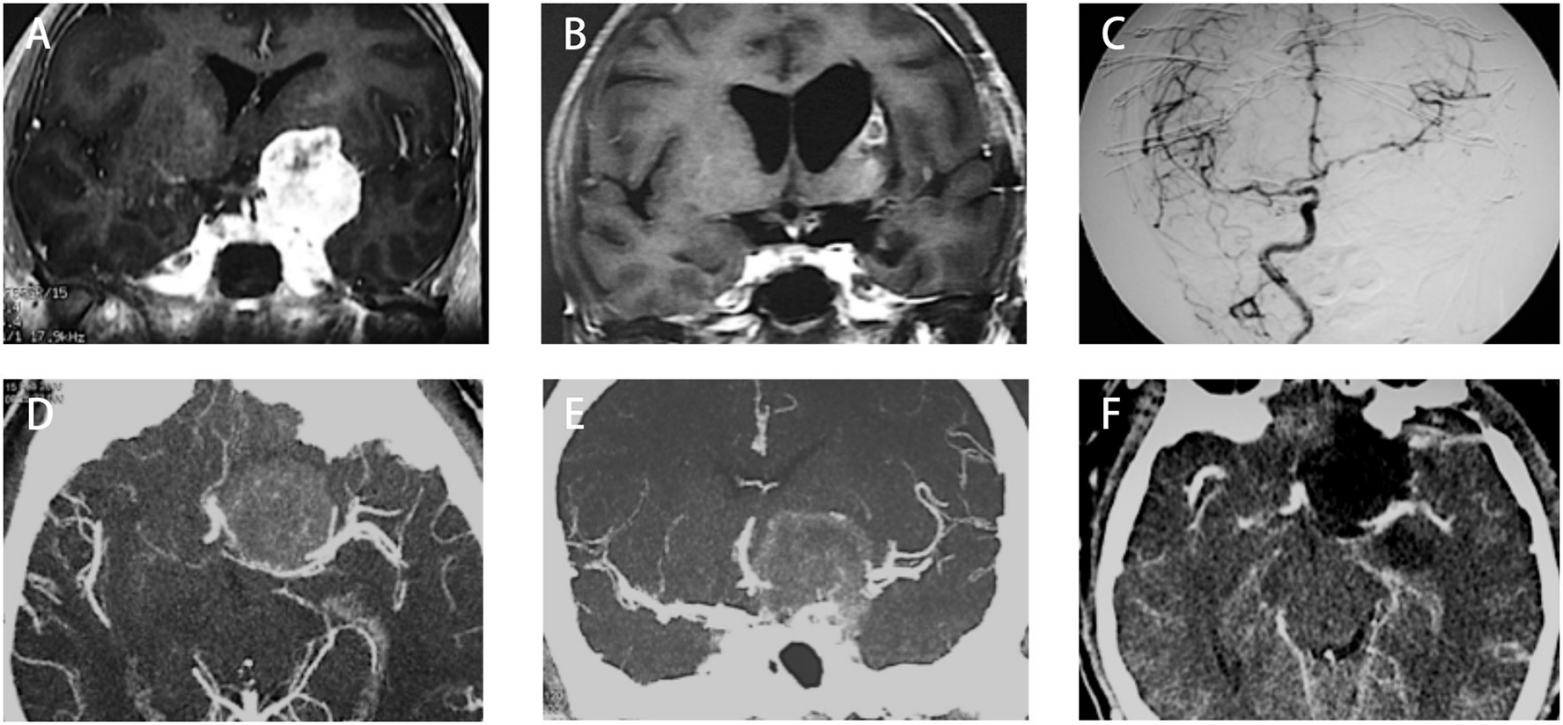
Two cases with anterior clinoidal meningiomas (ACMs) that experienced intraoperative rupture of internal carotid artery (ICA). (A) Preoperative coronal MRI showed a type I ACM, which involved the supraclinoidal part of the left ICA. (B) Postoperative coronal MRI showed gross-total resection of tumor, with a small infarction near the left side lateral ventricle, indicating the occlusion of perforators supplying the head of the caudle nuclei. (C) Postoperative angiography showed complete occlusion of the left ICA. (D,E) Preoperative CT angiography showed a type III ACM, which engulfs the bifurcation of the left ICA. (F) Postoperative CT angiography showed patent branches of the ICA with gross total removal of the tumor.
